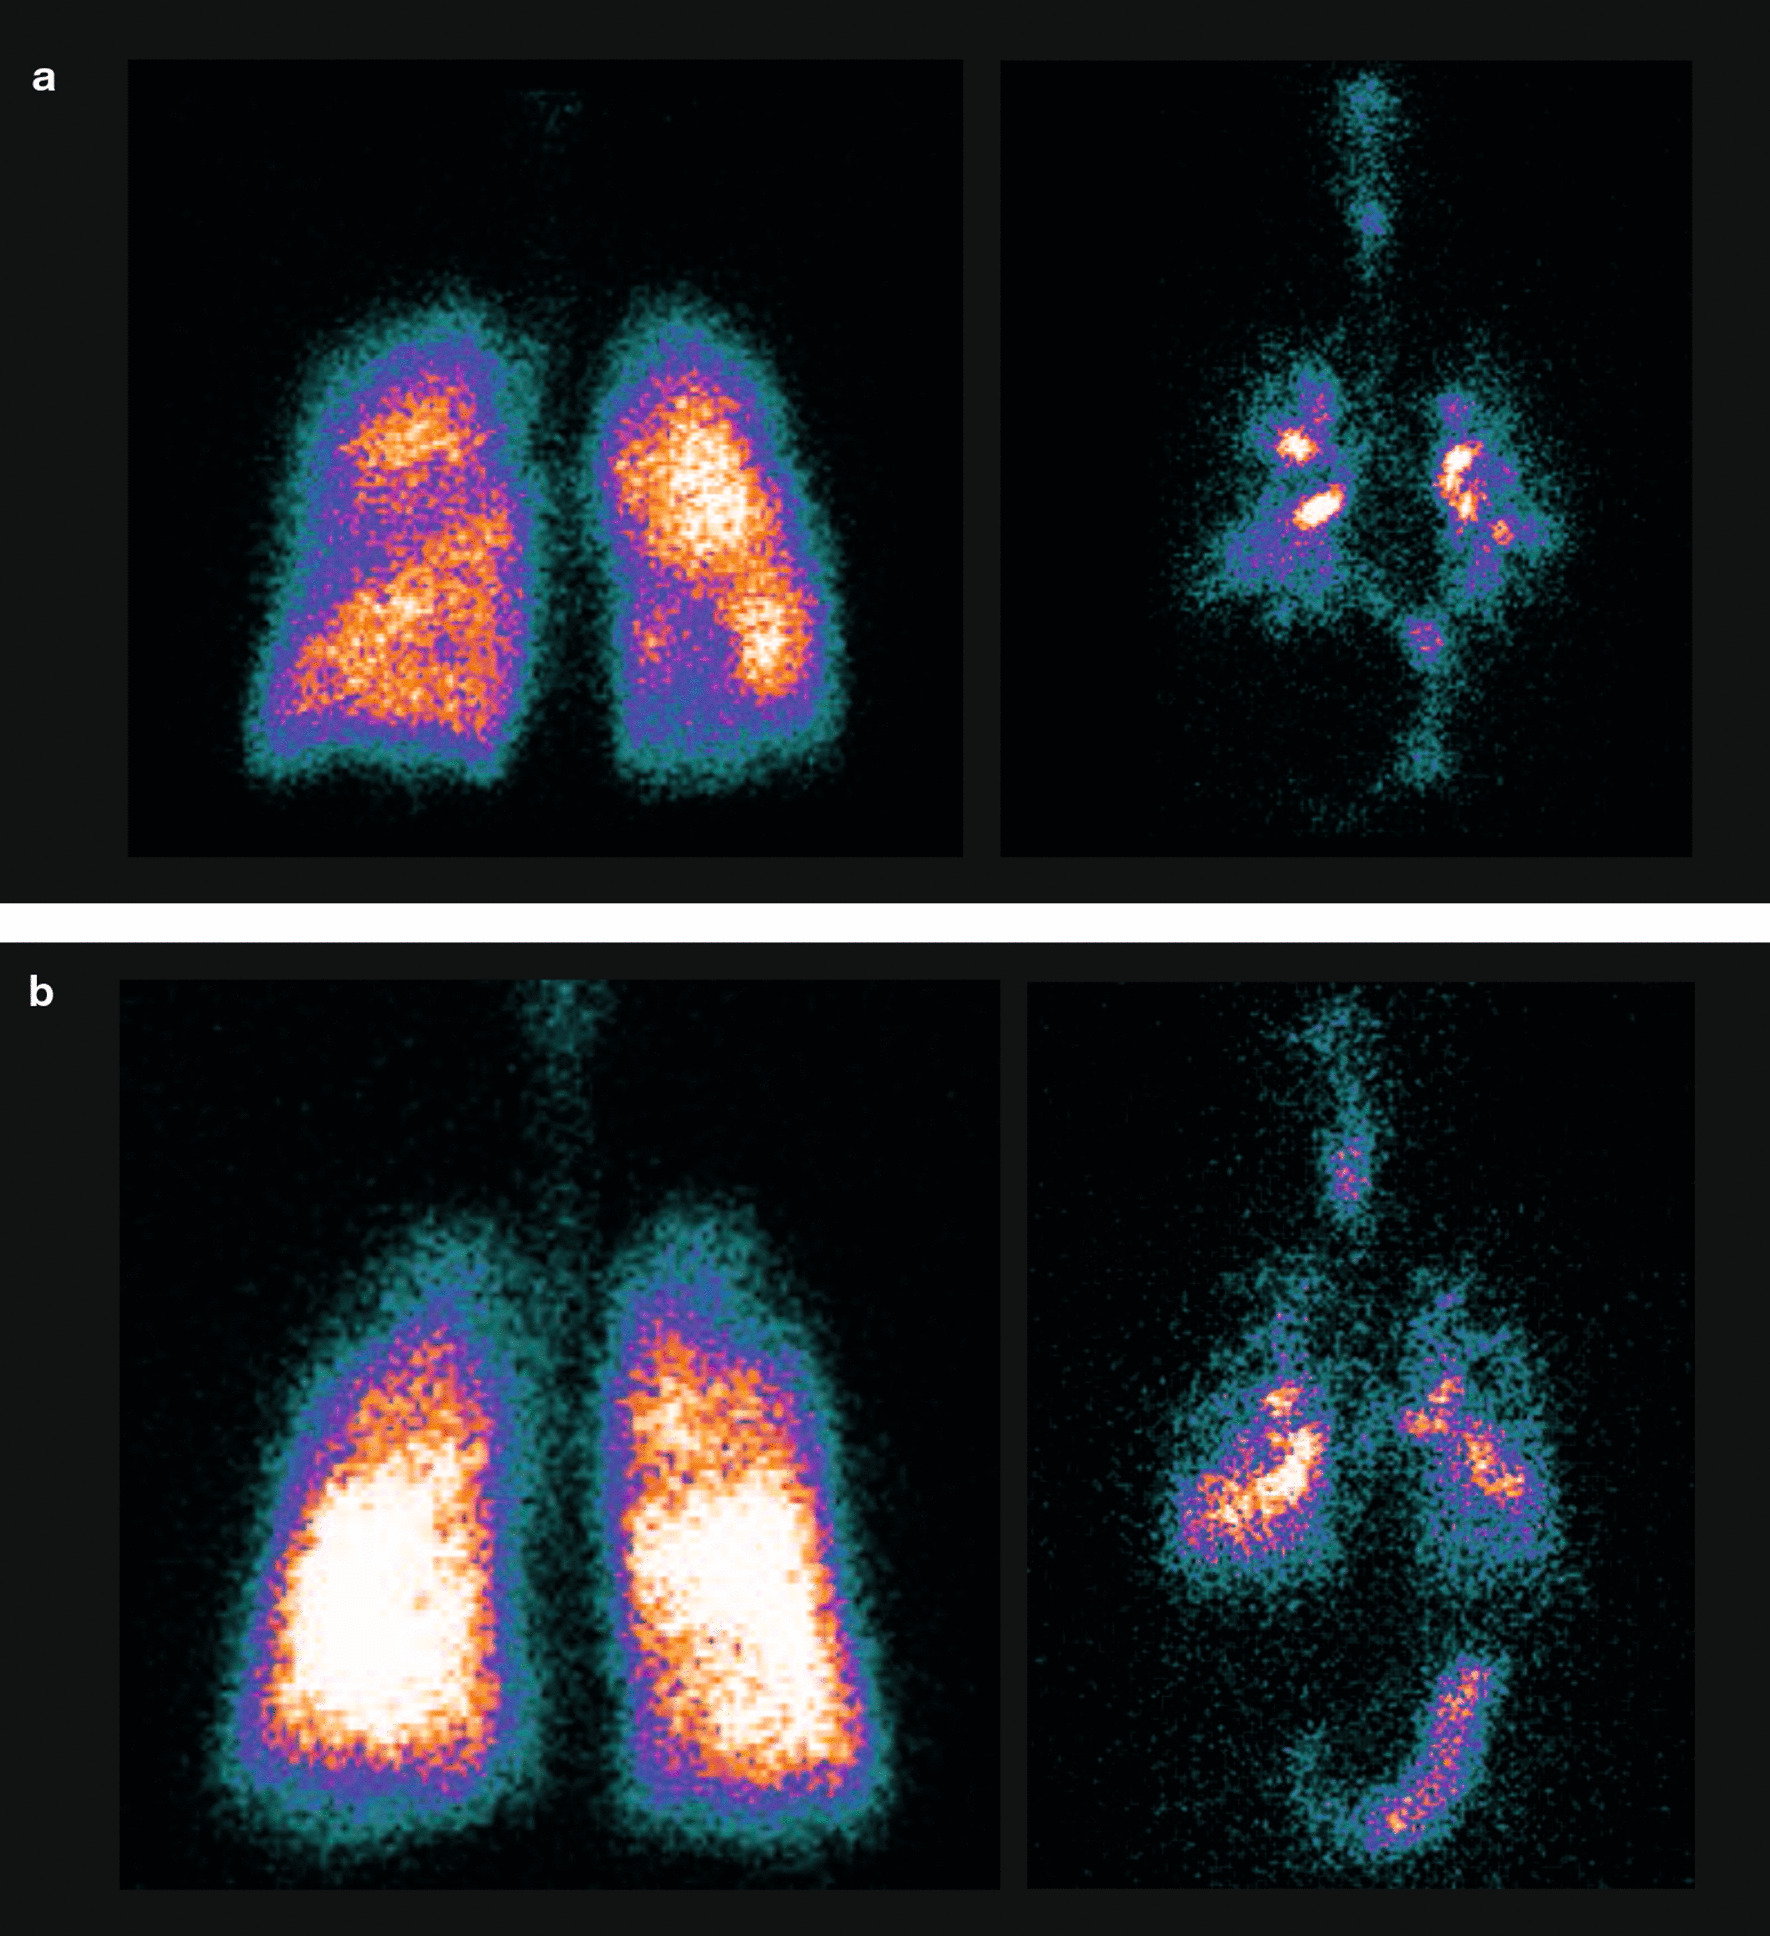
Representative gamma scintigraphy posterior view images of ^81m^Kr gas ventilation (left) and lung deposition of BGF (right) in a patient with a moderate COPD and a patient with b severe/very severe COPD (PP analysis set). ^*81m*^*Kr* Krypton-81 m, *BGF* budesonide/glycopyrrolate/formoterol fumarate, *COPD* chronic obstructive pulmonary disease, *PP* per protocol
